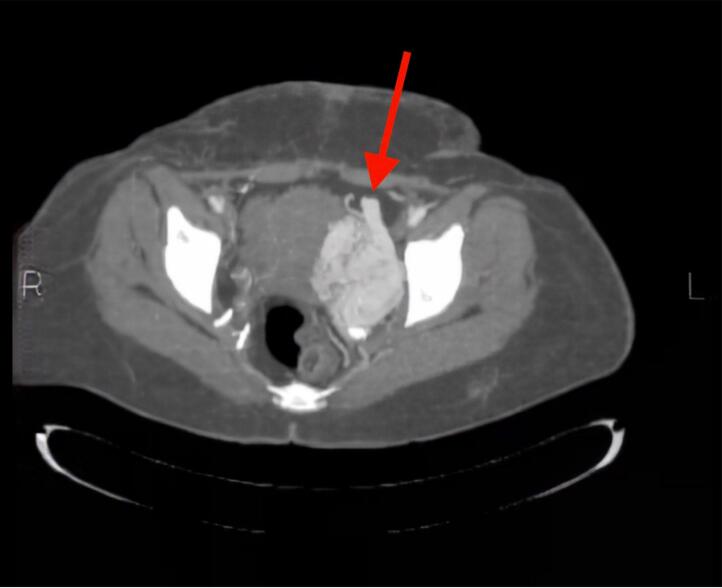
Abdominopelvic CT scan revealing a vascular mass in the left lateral pelvis that extended to the bladder neck highly suggestive of an AVM.
